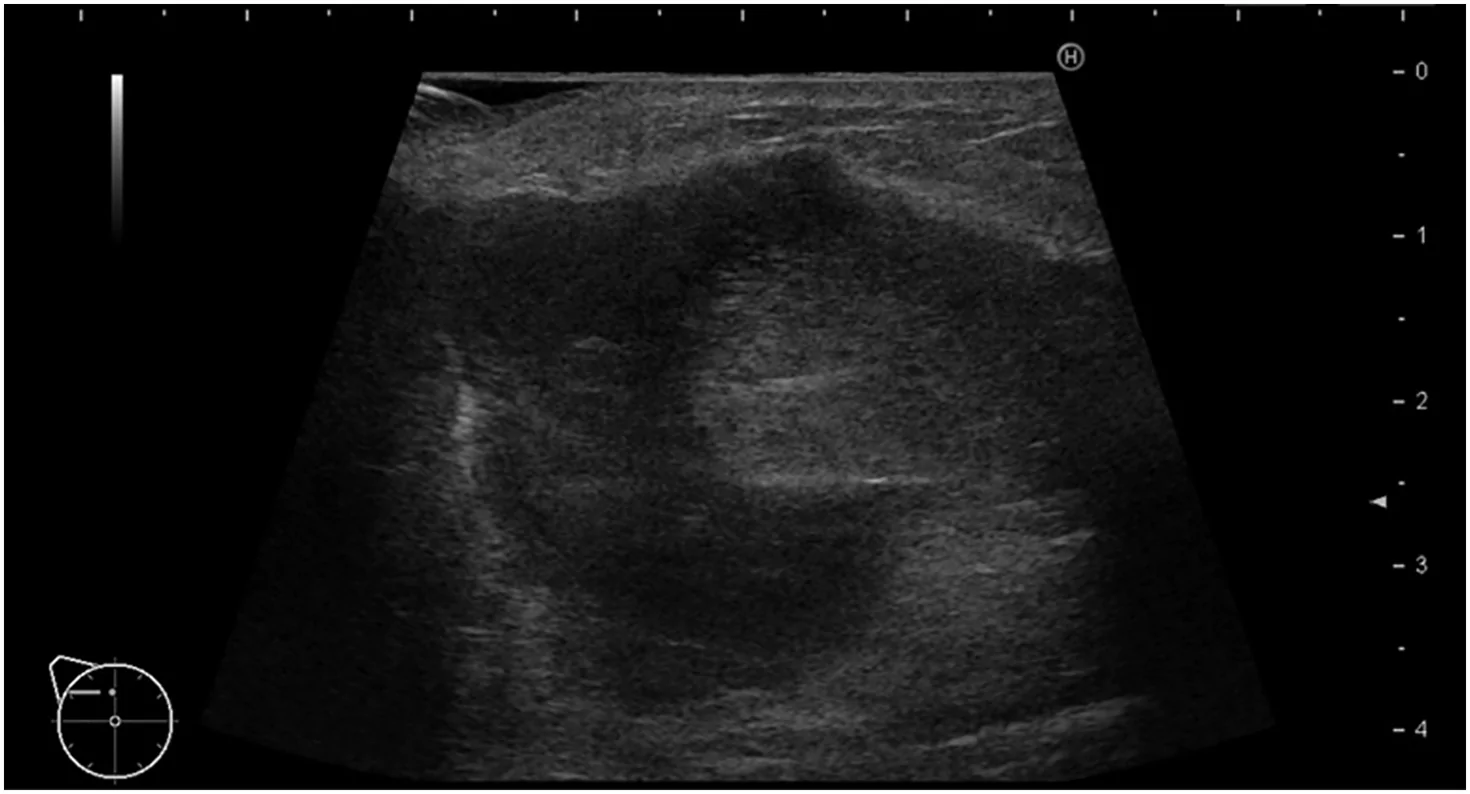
Breast ultrasonography findings. A lobulated mass measuring 40 × 30 × 50 mm was detected in the right breast. The mass contained both cystic and solid components, and intratumoral hemorrhage was suspected.
